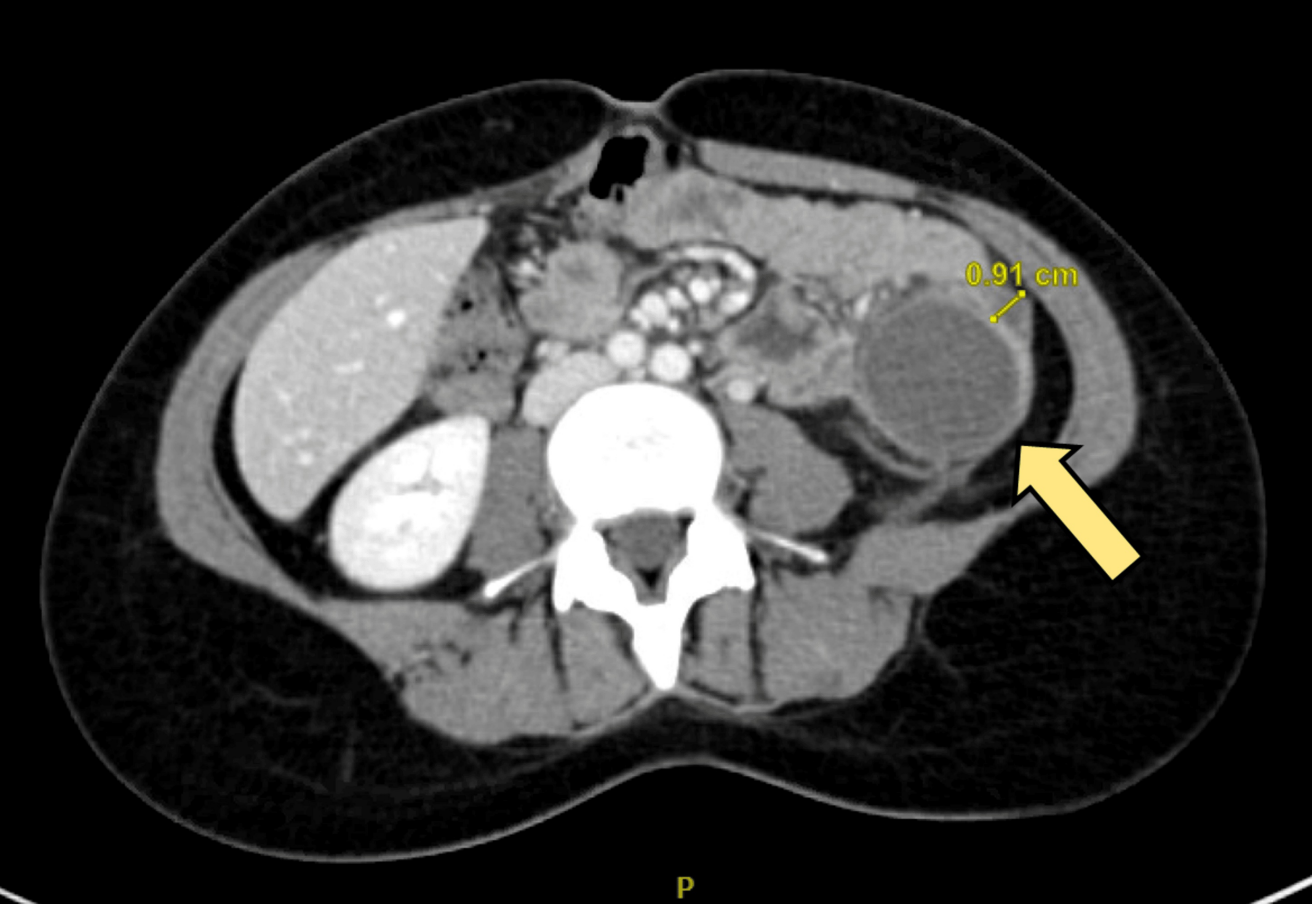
Axial view CT scan showing left sided colonic mass The yellow arrow is indicating the left-sided colonic mass. The 0.91 cm measurement indicates the thickness of the wall of the mass.
